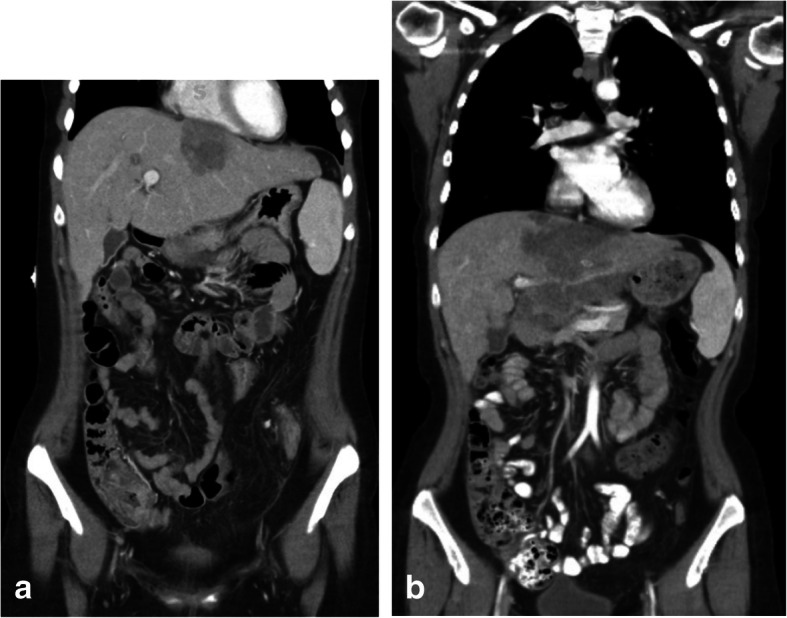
Radiographic evaluation of progression of disease. a Initial progression after 14 cycles of etoposide + methotrexate + actinomycin D ± cyclophosphamide + vincristine. b Further progression after five cycles of Folinic acid + fluorouracil + oxaliplatin + bevacizumab
